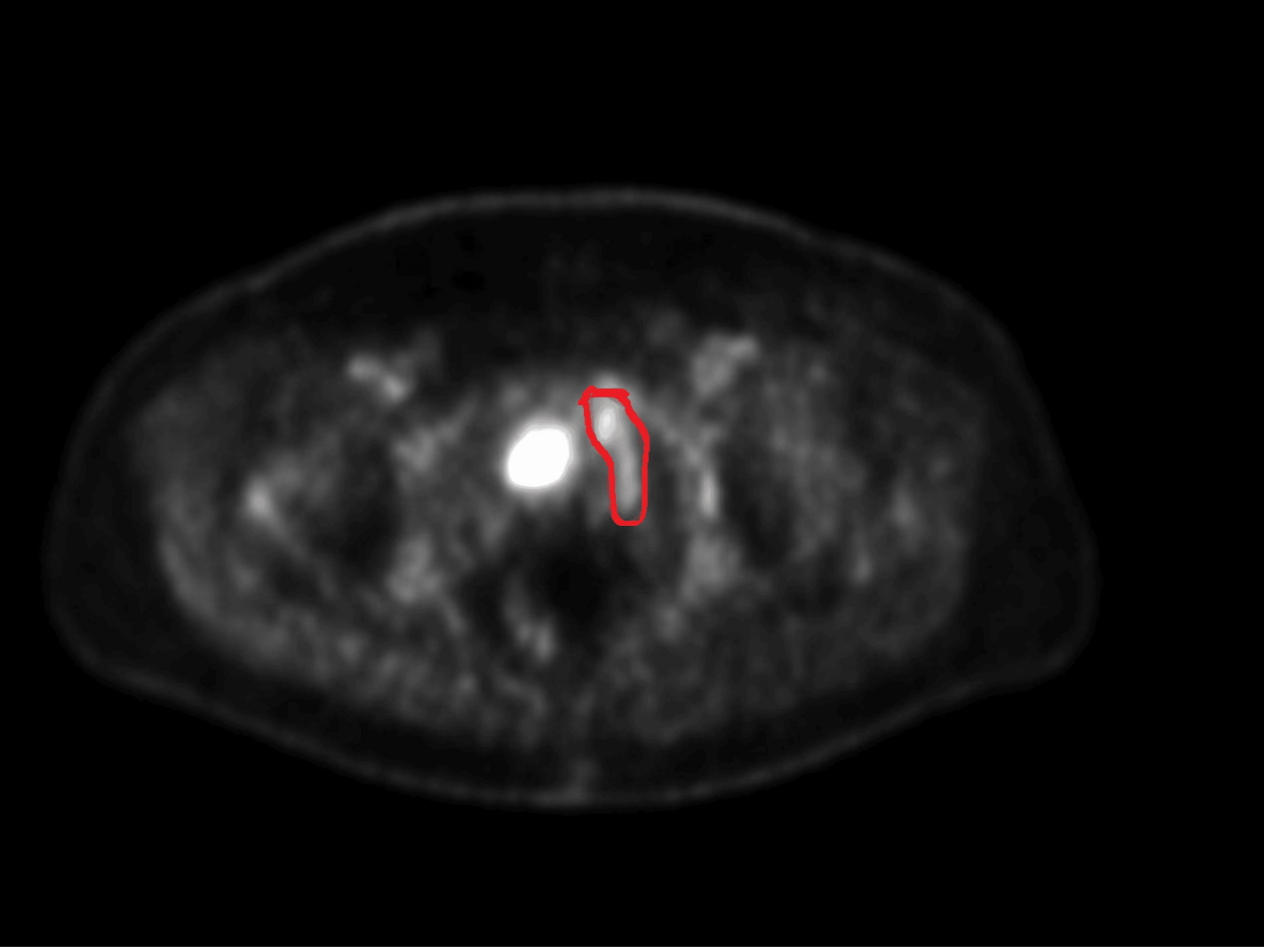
FDG PET-CT scan (February 2025) Axial PET image shows moderate, focal fluorodeoxyglucose (FDG) uptake (metabolic activity) in the left pelvic region (outlined in red), corresponding to the new mass identified on the MRI and raising suspicion for a metabolically active process.
